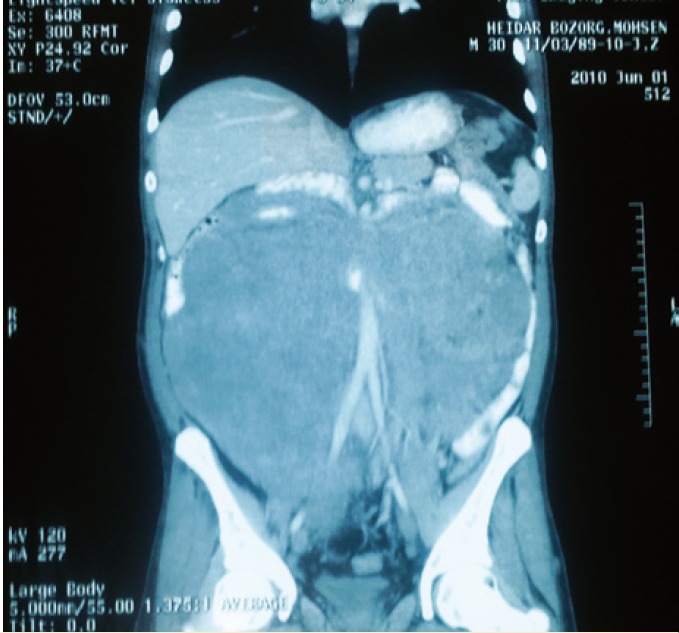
Preoperative abdominal computed tomography of the patient
